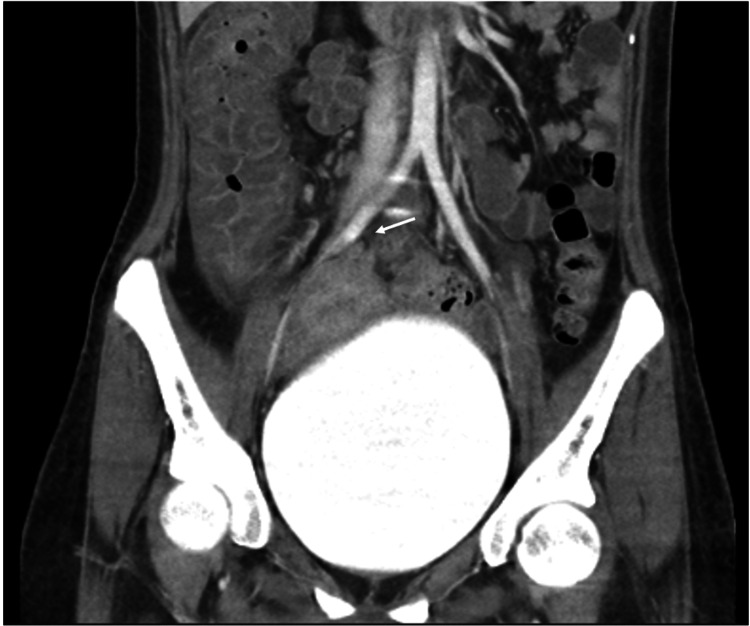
Computed tomography scan demonstrating thrombosis of the left common iliac vein (white arrow).
